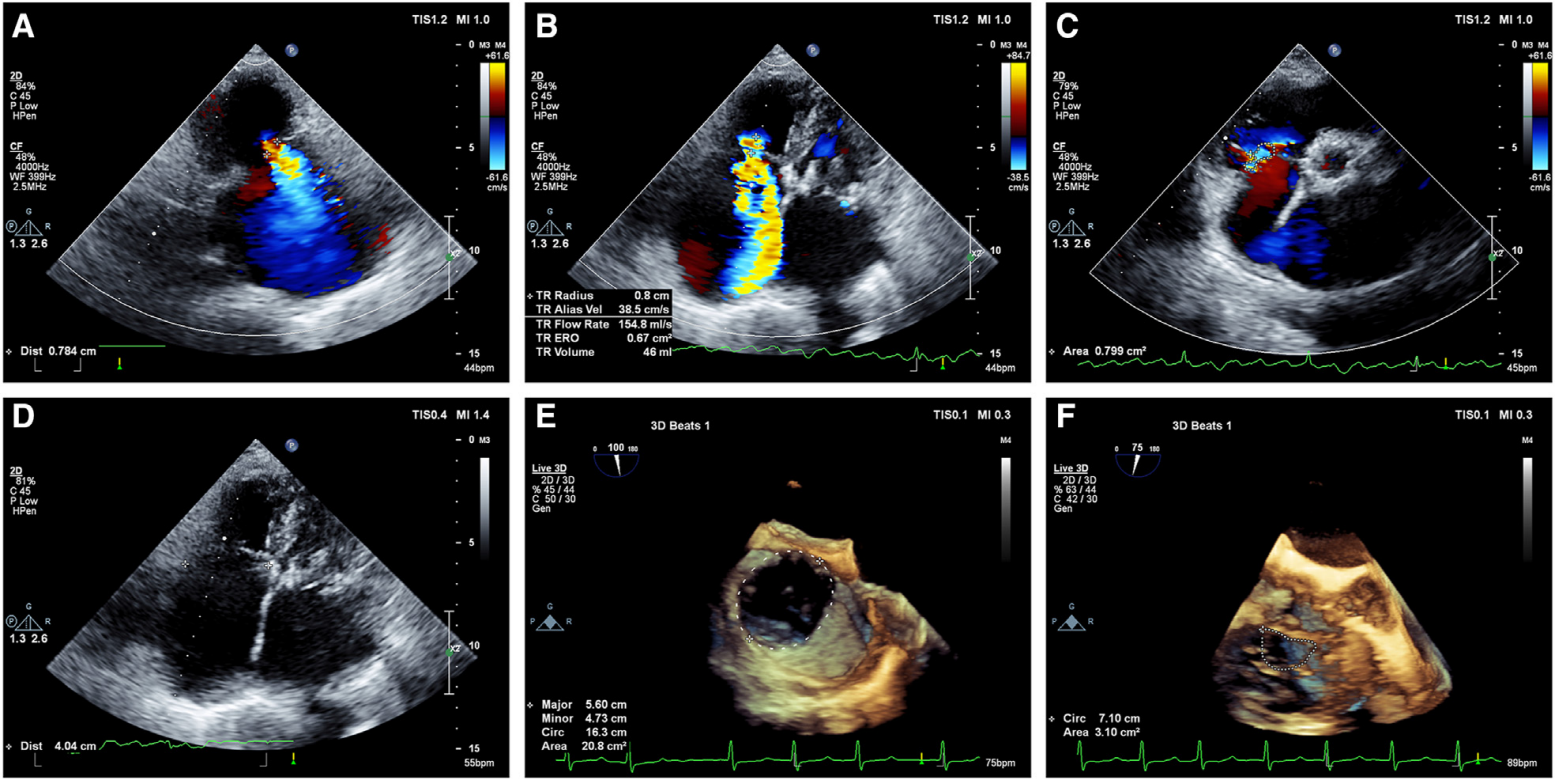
Preprocedural TTE and TEE (A) TR vena contracta width is measured from TTE (width, 7.8 mm). (B) PISA estimated Doppler volume method EROA and regurgitant volume are measured from TTE (EROA, 67 mm^2^; regurgitant volume, 46 mL). (C) The vena contracta area is measured from TTE (area, 79.9 mm^2^). (D) The septolateral diameter of the tricuspid valve annulus is measured from TTE (diameter, 4.0 cm). (E) The maximum circumference and area of the tricuspid annulus are measured from 3D TEE in diastole (circumference, 16.3 cm; area, 20.8 cm^2^). (F) The gap shape is shown from 3D TEE in systole (gap area, 3.1 cm^2^). 3D = 3-dimensional; EROA = effective regurgitant orifice area; PISA = proximal isovelocity surface area; TEE = transesophageal echocardiography; TR = tricuspid regurgitation; TTE = transthoracic echocardiography.
